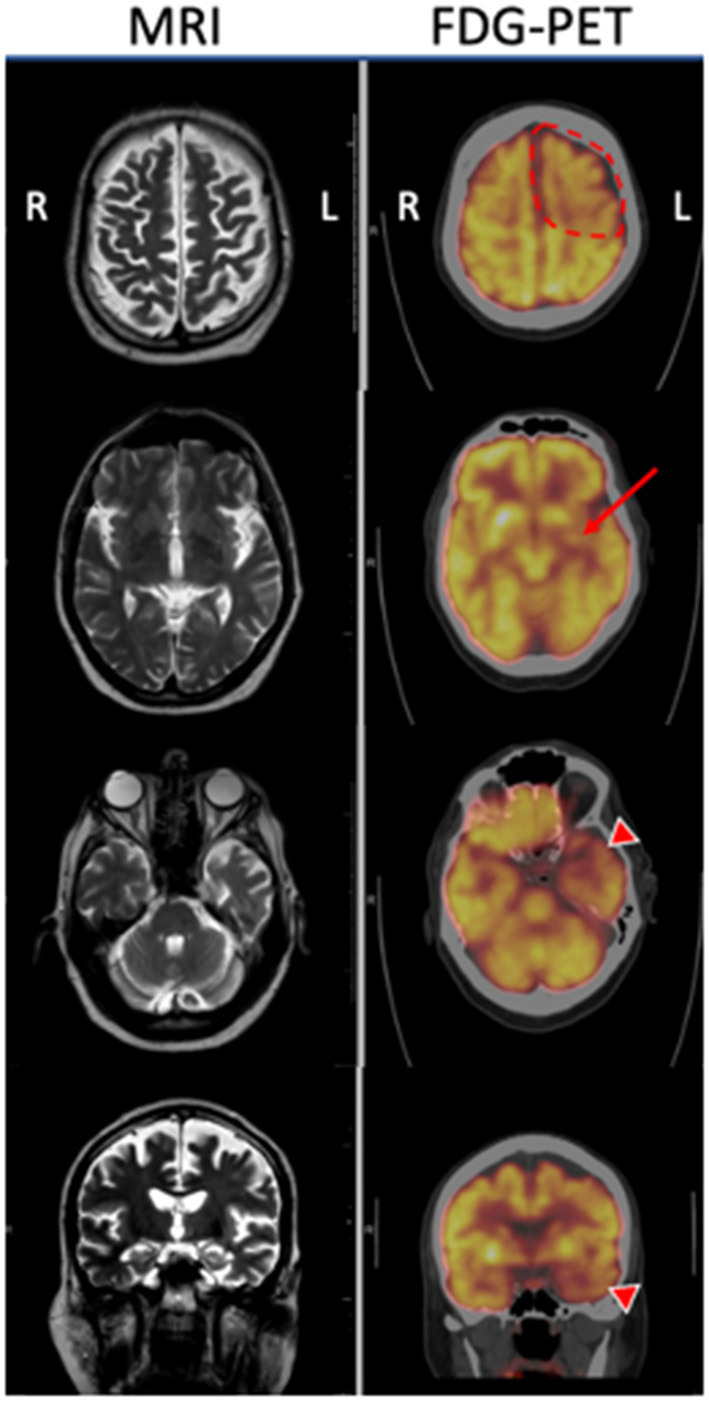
MRI T2 sequences and corresponding FDG-PET imaging of the proband at three axial levels and a coronal image through the hippocampi. MRI demonstrates generalized cortical atrophy of the left hemisphere, especially evident within the left anterior temporal lobe. FDG-PET reveals hypometabolism of left frontal lobe (dotted line) that was more prominent than the right, in addition to the left putamen (arrow), and left temporal lobe (arrowheads) compared with the corresponding regions on the right.
